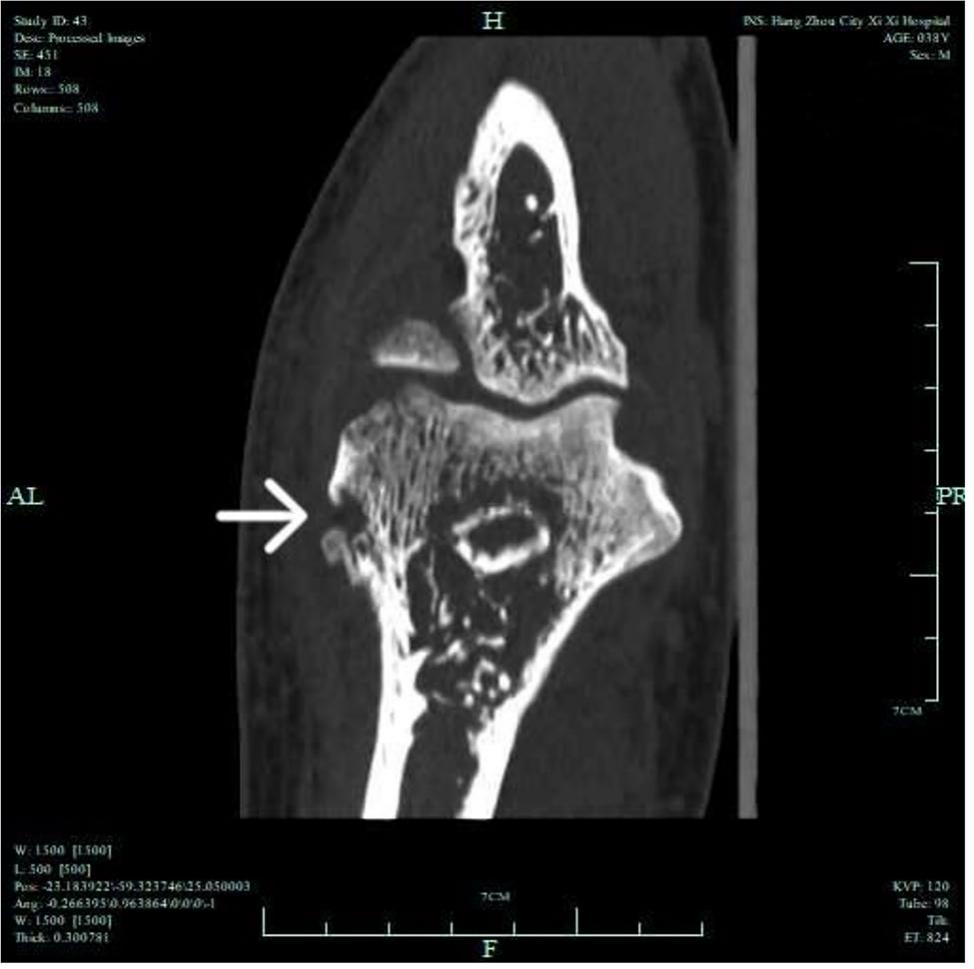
CT scan of the left elbow joint exhibited a reduced density area of worm erosion in the lower end of the humerus and the olecranon cortex of the ulna. The trabecular structure was disorganized, the left elbow was properly positioned, surrounding soft tissue was swollen, and fluid was visible in the joint space
